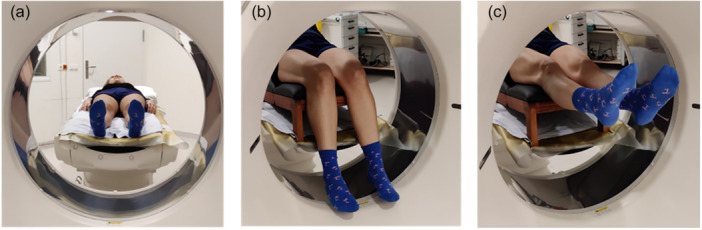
Overview of the computed tomography (CT) scanning protocol. (a) A static CT scan was performed with subjects in a supine position. (b, c) Dynamic CT scans were acquired with subjects in a semiseated position at the end of the scanner table while performing a flexion‐extension‐flexion movement from 90° to full extension with both legs simultaneously over 10 s. Adapted from Dunning et al. [8], licensed under CC BY 4.0.
